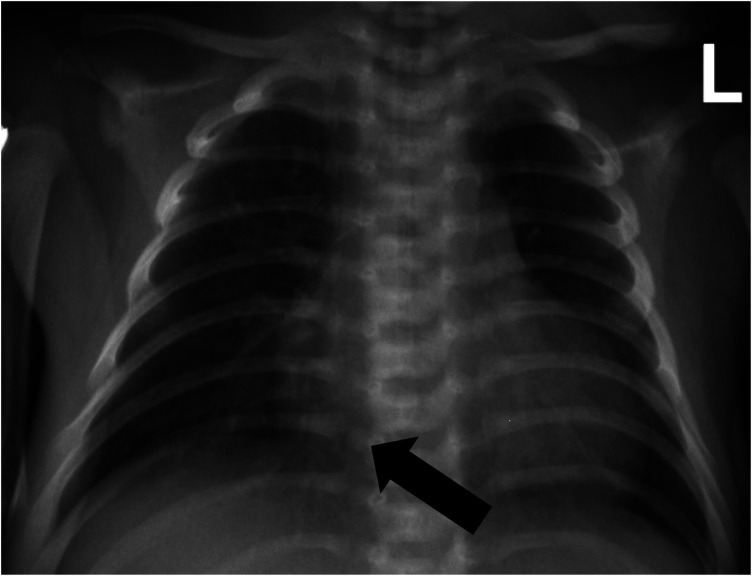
Chest x-ray showing improved exudative lesions in both lungs.
